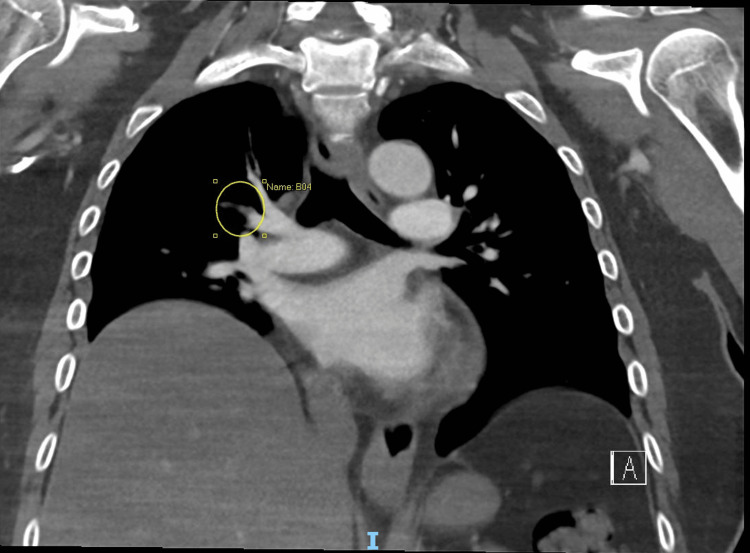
Computed tomography pulmonary angiography (CTPA), coronal view. Filling defects were noted around the subsegmental branches of the pulmonary arteries in the upper lobes of the lungs (yellow circle).
